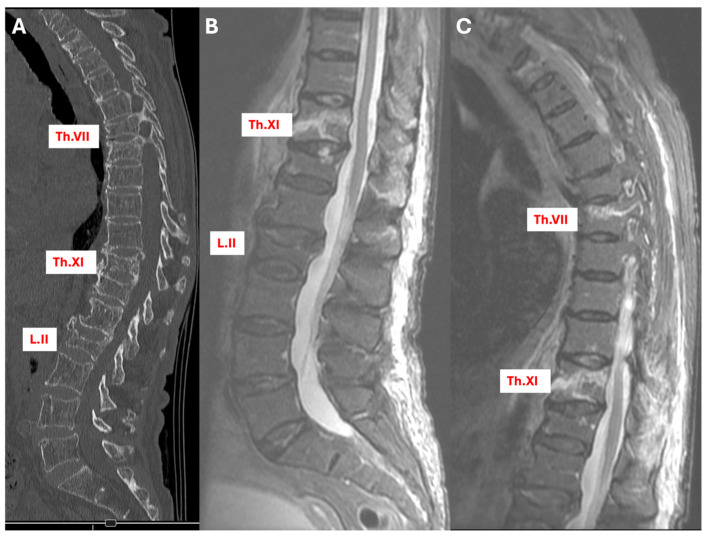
Sagittal section views of a patient with multiple fractures. (A) CT scan, (B) STIR MRI in the lumbar region, and (C) STIR MRI in the thoracic region, with a fresh fracture indication at Th.VII.
